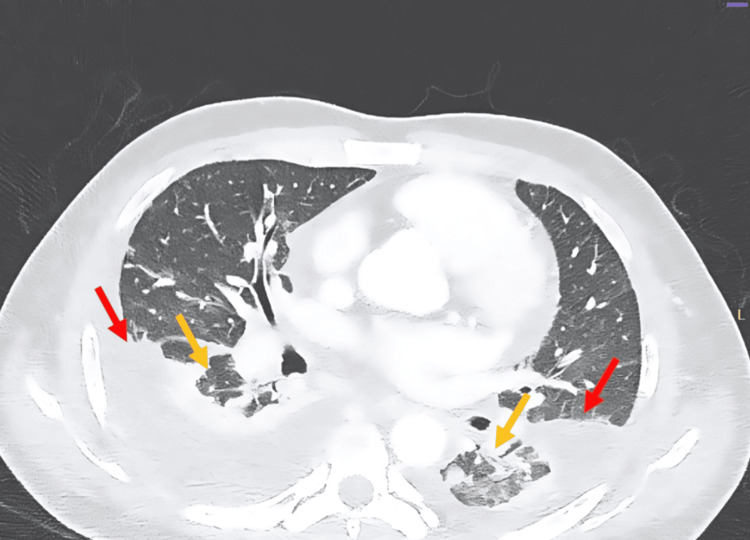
CT of the chest showing bilateral rounded areas of low attenuation with surrounding consolidation (yellow arrows) with associated parapneumonic effusions (red arrows) CT: computed tomography
